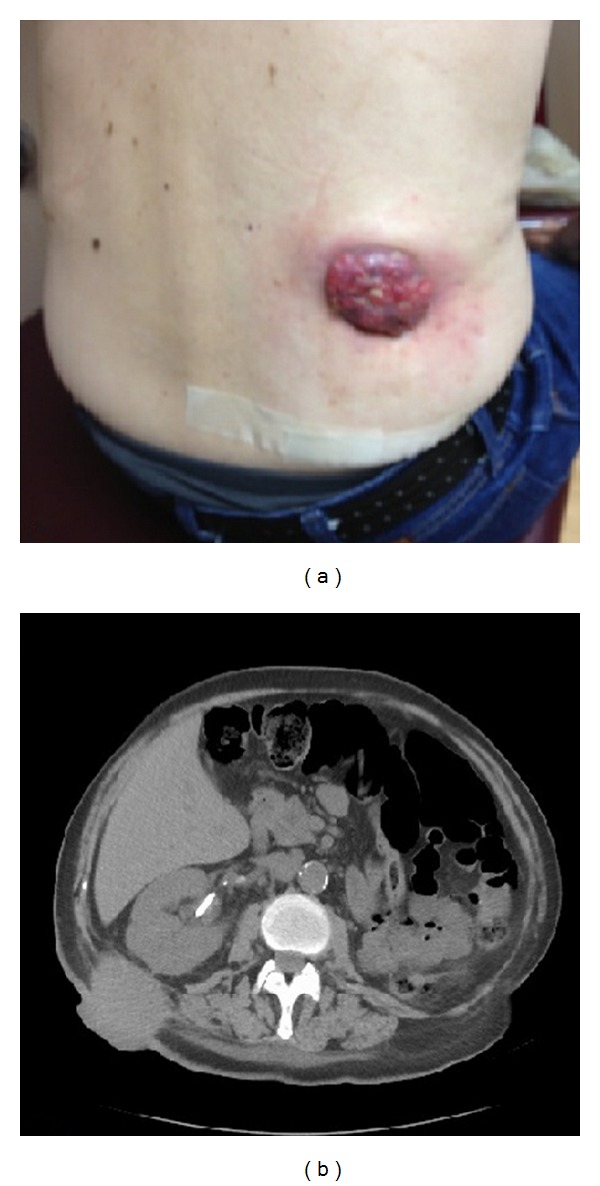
(a) Soft tissue mass at previous traumatically removed nephrostomy site, (b) CT scan revealing extent of the soft tissue mass.
